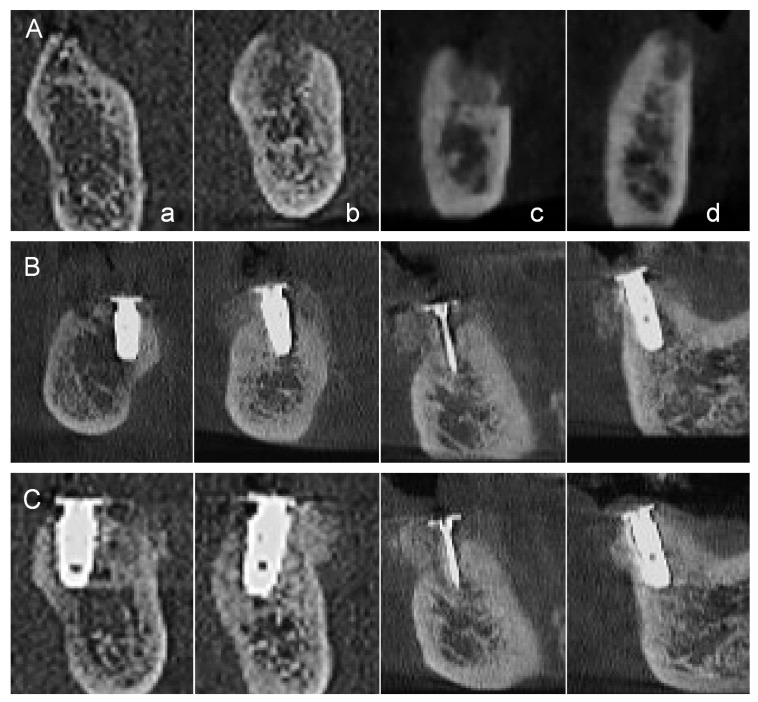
Serial cross-sectional CBCT images. Tooth positions: a—second molar; b—first molar; c—second premolar (WHTPS site); d—first premolar. (A) Preoperative scan showing vertical alveolar bone deficiency at the edentulous site. (B) Immediate postoperative scan following vertical ridge augmentation using a wide-head tent pole screw (WHTPS) and particulate bone graft. (C) Scan at 4 months postoperatively, taken on the day of uncovering, showing stable graft volume and cortical bone formation.
